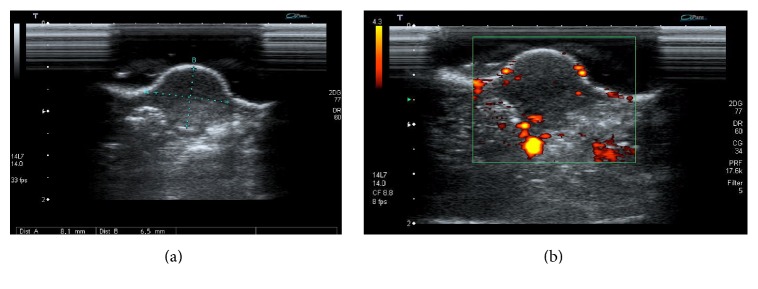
(a) Tumor volume evaluated in B-mode by ultrasonography. (b) Example of Doppler imaging for the assessment of the number of intratumor vessels.
